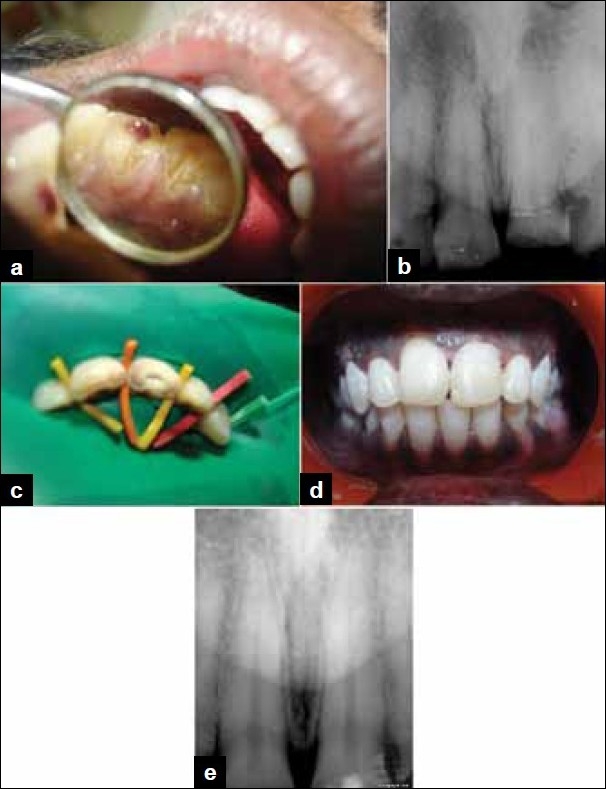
(a) Preoperative photograph showing Ellis class III fracture in 21 with pulp exposure, (b) Preoperative radiograph revealing fracture in 21 with pulp involvement, (c) Partial pulpotomy performed in 21 and MTA placed over the exposed pulp, (d) Postoperative photograph, (e) Postoperative radiograph after two years showing no radiographic changes
